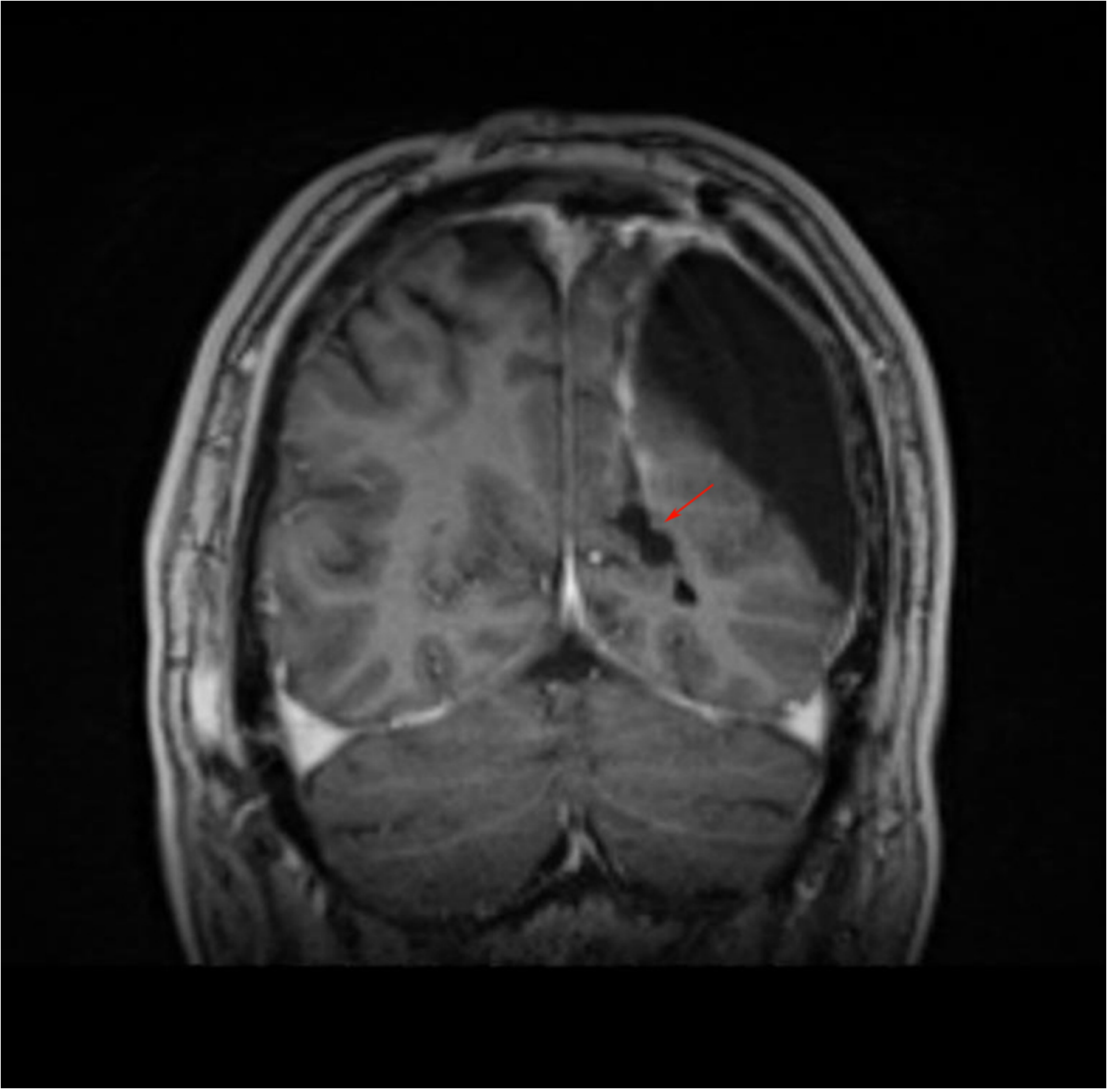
Postoperative coronal T1-weighted MRI. Red arrows point at the location where the circular outlet of the ringed vascular graft can be seen
